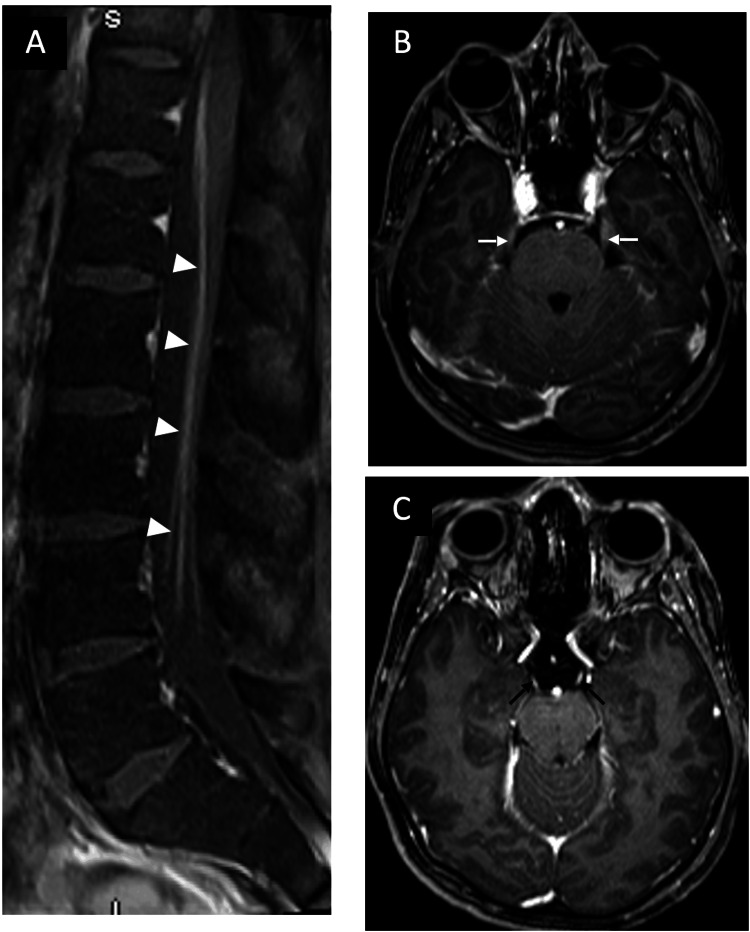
Cranial nerve and nerve root enhancement A, sagittal post-contrast T1-weighted image of the lumbar spine demonstrates anterior cauda equina nerve root enhancement (arrowheads). B and C, axial post-contrast T1-weighted images demonstrate trigeminal (white arrows) and oculomotor (black arrows) nerve enhancement, respectively.
